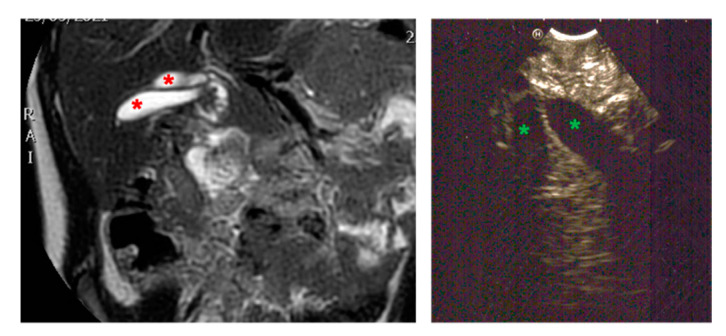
A duplicated gallbladder is seen on MRI scans (red asterisks) and intraoperative endoscopic US (green asterisks) in patient n. 7. The boy came to our attention after prenatal detection of the double gallbladder and annular pancreas. Cholangio MR showed two gallbladders that merged at the level of the proximal cystic duct and a cystic duodenal duplication between the second and third duodenum. The endoscopy showed mild duodenal stenosis below the first duodenum due to ab extrinsic compression by the annular pancreas. The US evaluation identified a normal choledochal channel (1.2 mm), an annular pancreas, and a cystic duodenal duplication (as shown in Figure 1) in the second duodenum. Biliopancreatic ducts ended up more distally. The gallbladder appeared duplicated with two cystic ducts that merged with the choledocus. No biliary dilatations were identified.
